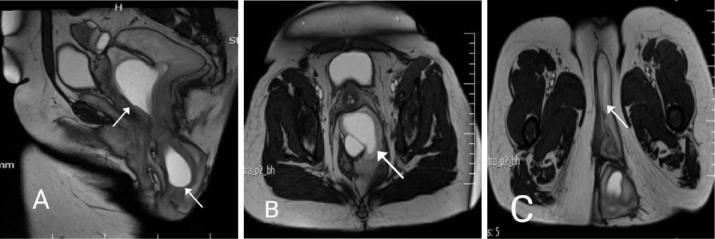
Pre-operation imaging A) Sagittal plane of pelvic MRI (T2-weighted MRI), indicating a large mass with longitudinal extension from the posterior of the uterus toward the ischiorectal and ischioanal spaces with a displacement of adjacent organs. B) Transverse plane of pelvic MRI indicates a left pararectal mass that causes displacement of the rectum to the right and is located behind the vagina. C) This MRI plane shows the involvement of the left side of the vagina (white arrow).
